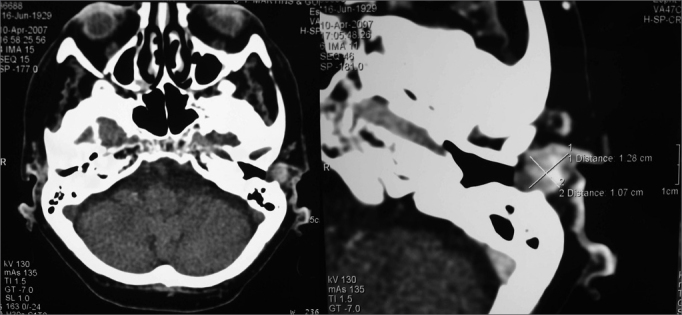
Adenoid Cystic Carcinoma in Left External Ear Canal - Axial view CT scans of temporal bones (with and without contrast enhancement)
